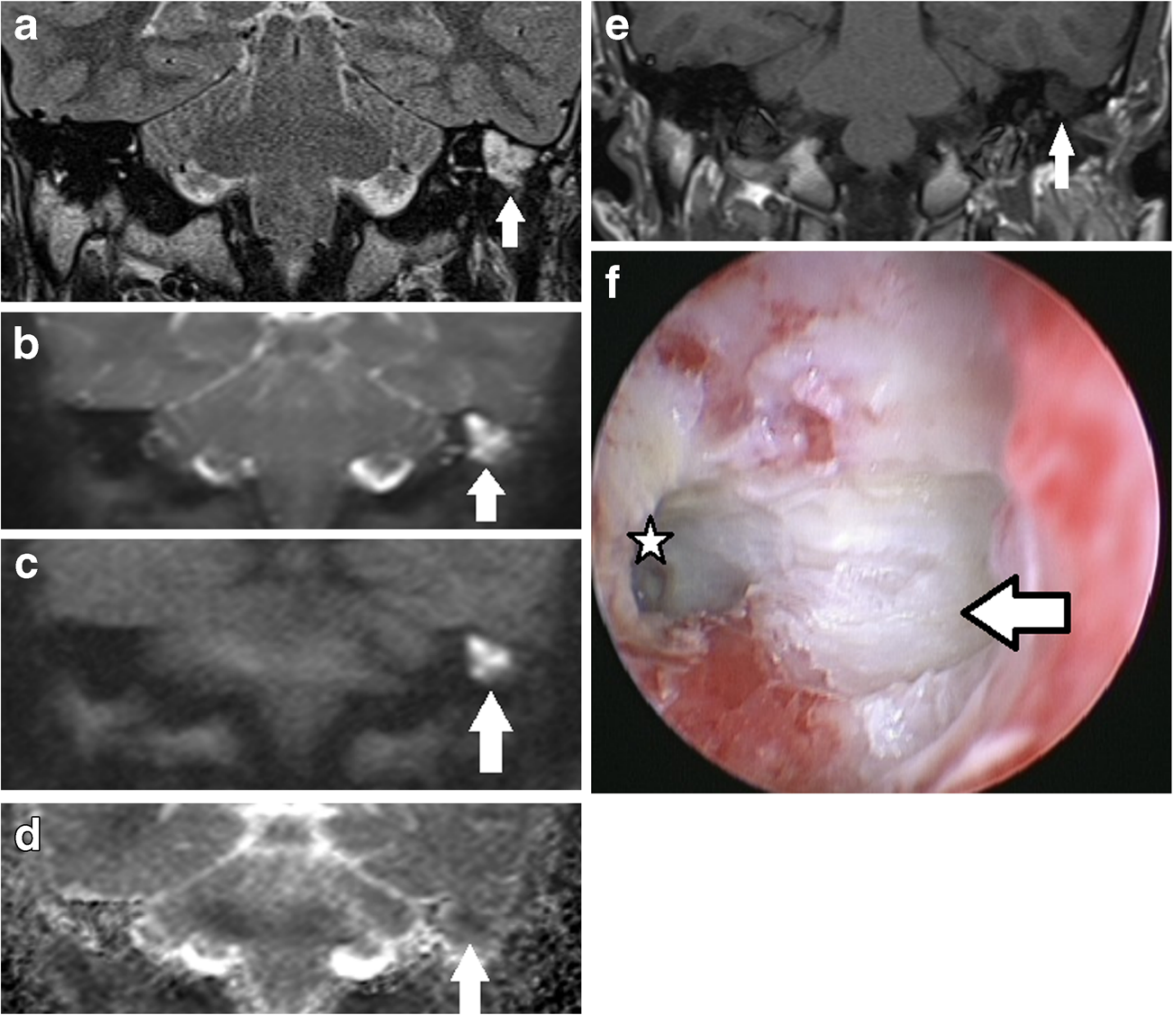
Typical MRI characteristics of post-operative cholesteatoma. Coronal images through the left mastoid remnant shows the lesion (*white arrow*) as high signal on a the T2 weighted image, b DWI b0 image, and c DWI b1000 image and a low signal on the d ADC map and e non-contrast enhanced T1 weighted image. f Surgery confirms the presence of the cholesteatoma. The keratin has been suctioned away showing the underlying white squamous epithelium lining the mastoid remnant (*white arrow*) and aditus (*star*)
